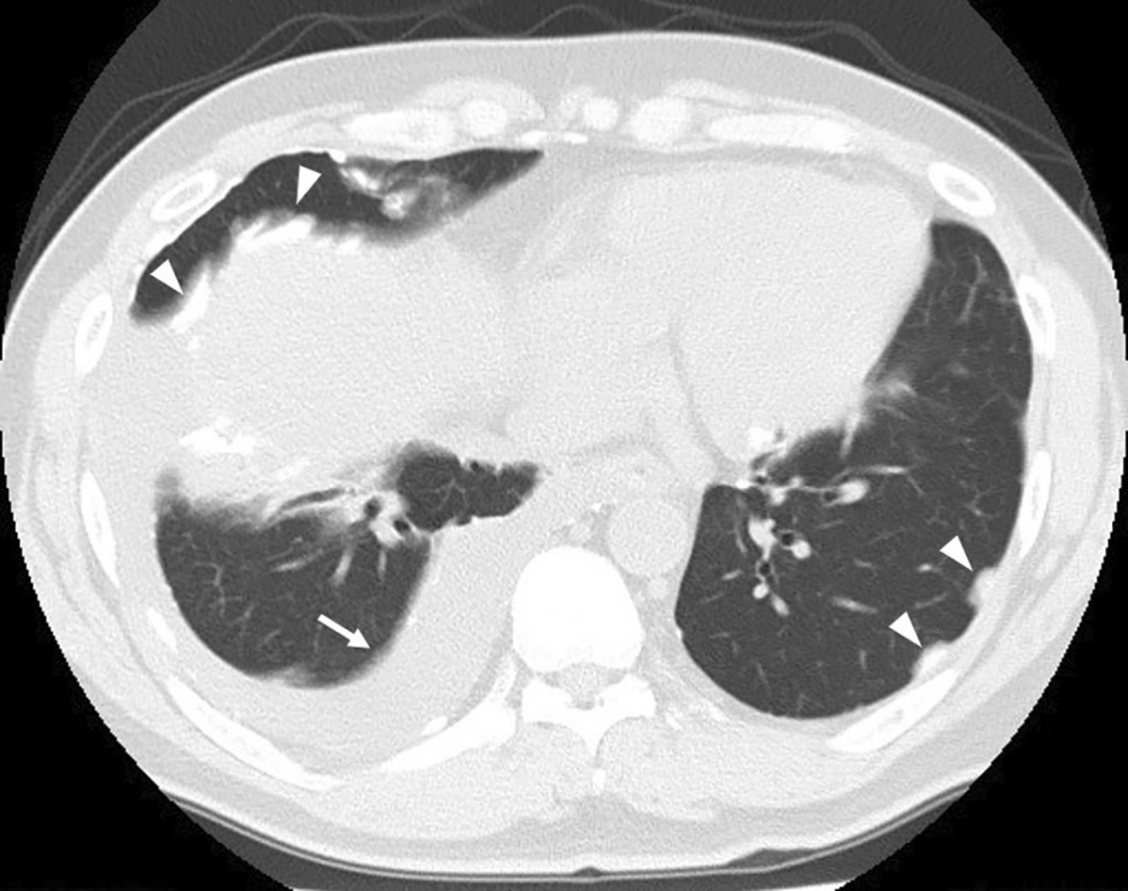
Computed tomography showing pleural dissemination (▲) and effusion (→).
